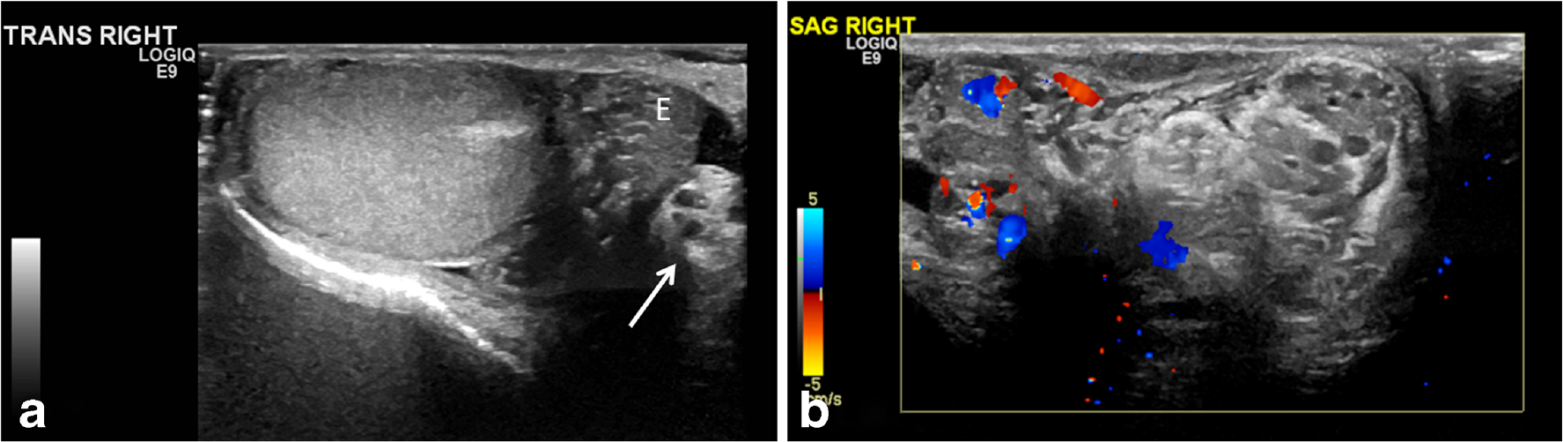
Intermittent torsion in a 17-year-old boy who presented with 5 h of acute right testicular pain after a game of football. He had experienced 6–7 similar episodes in the last 2 years where the pain had spontaneously resolved. Cremasteric reflex was absent on the right. a Gray-scale transverse US image of the right testis shows a redundant spermatic cord (*arrow*) occupying the medial half of the scrotal sac, with a mildly edematous epididymis (*E*) adjacent to it. The echogenic mediastinum testis faced medially instead of posterolaterally, which was concerning for altered testicular lie. b Color Doppler longitudinal image of the right scrotum shows excess and tortuous spermatic cord bunched up in the scrotal sac superior to the testis and formation of pseudomass, suggesting torsion of the spermatic cord. Note that this extratesticular pseudomass is not hyperemic and should not be confused with epididymitis. Orchiopexy was recommended; however the family chose to wait because his pain improved. Elective orchiopexy was performed 7 months later and bilateral bell clapper anomaly was noted; he was diagnosed with intermittent torsion
